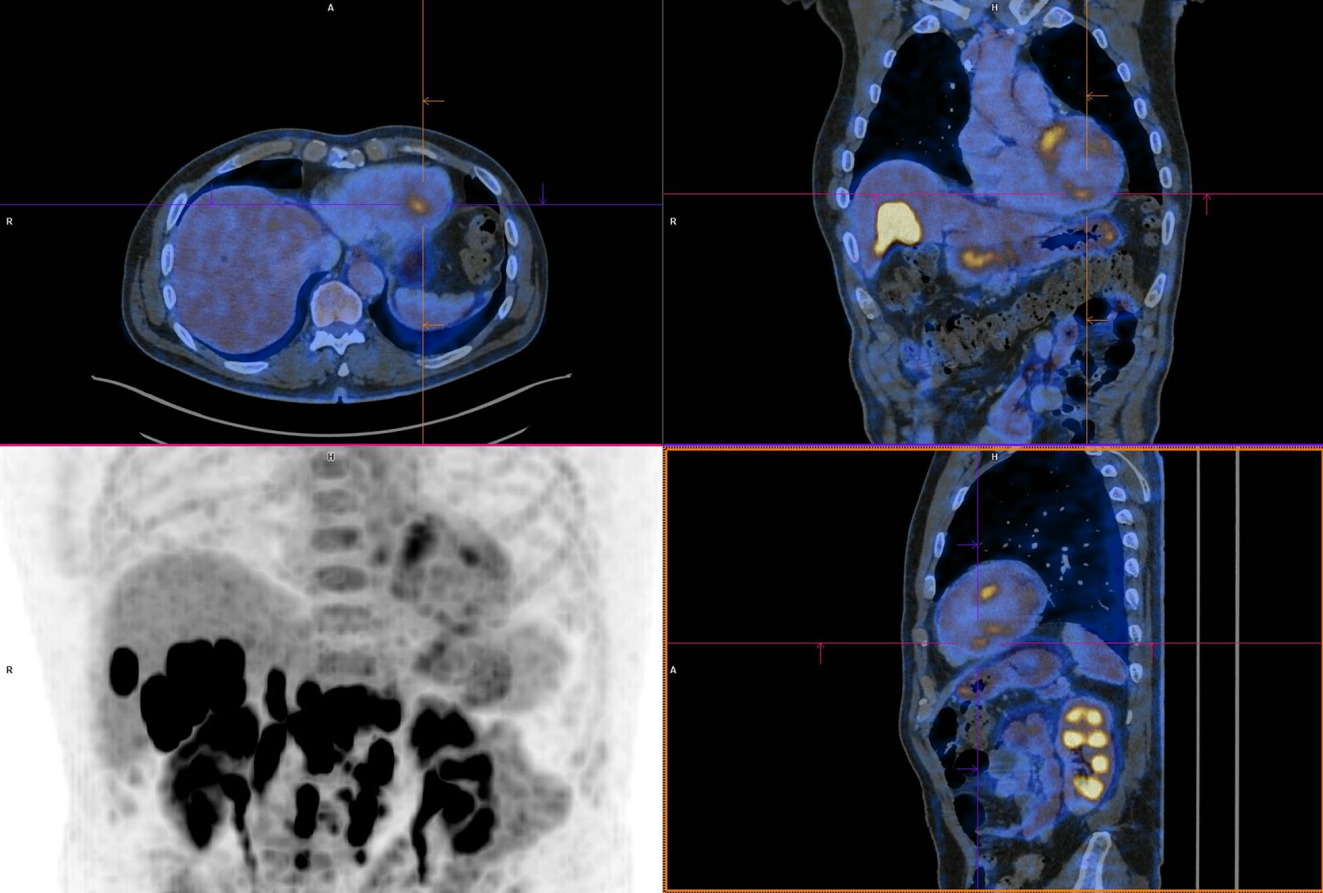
FDG-PET/CT depicting multiple zones of myocardial inflammation: basal septal, mid-anterior, and apical inferior
